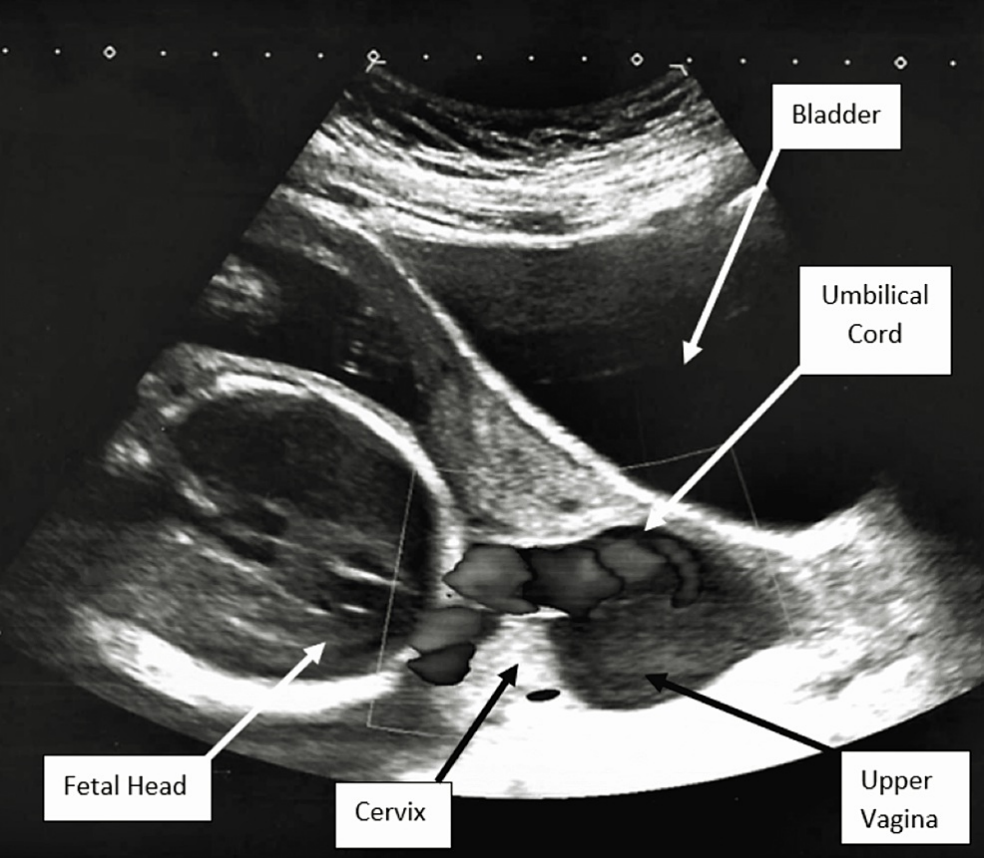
Transabdominal ultrasound identification of umbilical cord prolapse with intact membranes.
